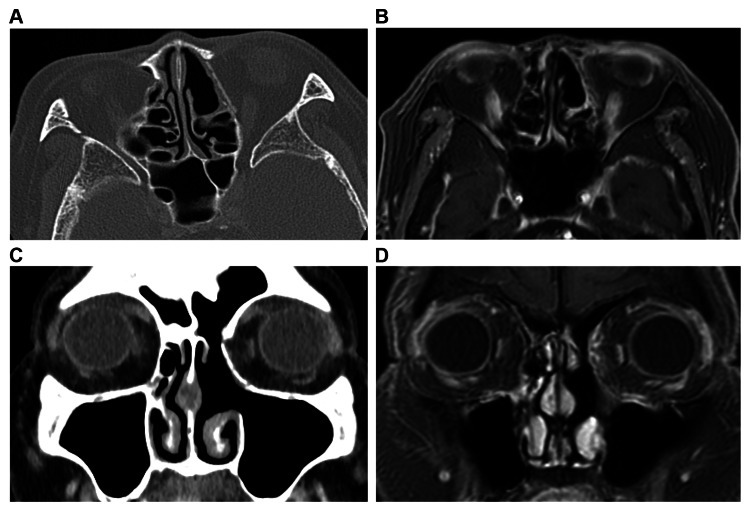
Postoperative CT/MRI scan: (A) CT axial image, (B) CT coronal image, (C) MRI axial T1-weighted image, and (D) MRI coronal T1-weighted image No evidence of postoperative recurrence is seen. CT: computed tomography; MRI: magnetic resonance imaging Source: Image of the patient showing the condition/feature. Informed consent for the use of this image was obtained from the patient.
